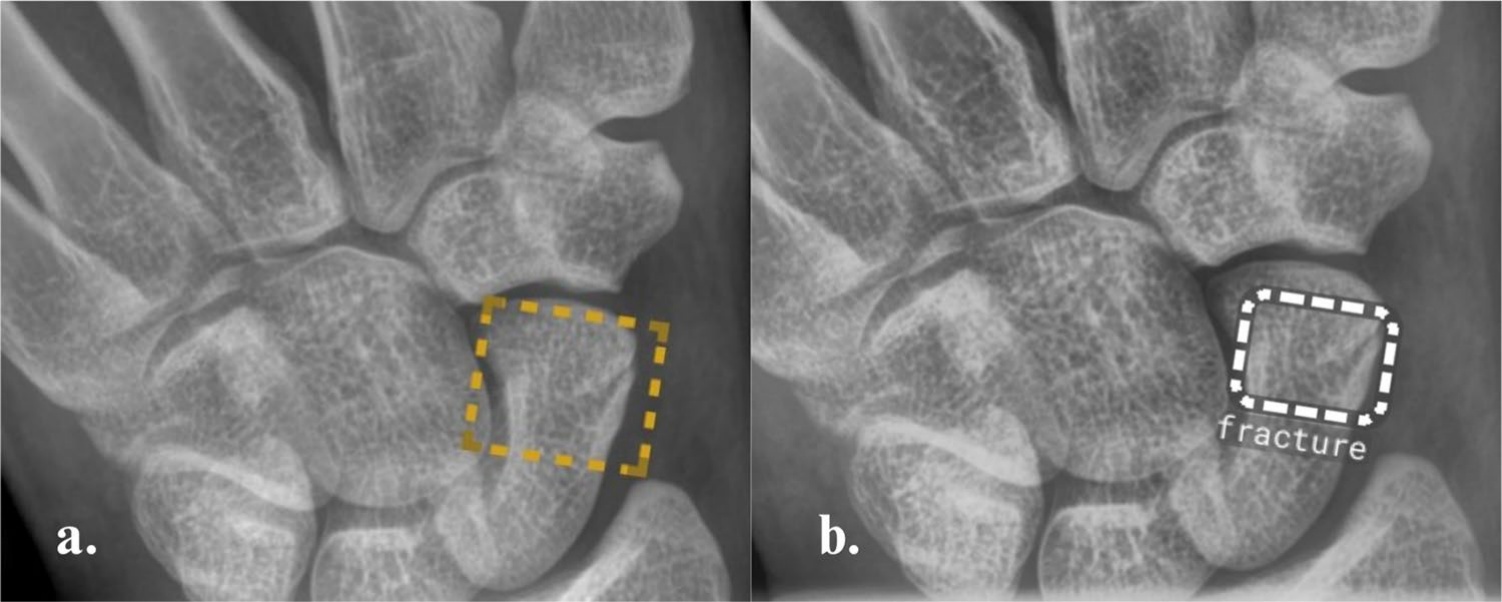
Posteroanterior radiograph of the wrist of a male patient. A scaphoid fracture is marked with a dashed bounding box, indicating a possible fracture (‘Doubt’), by the AI software SW1 (a) and SW2 (b)
